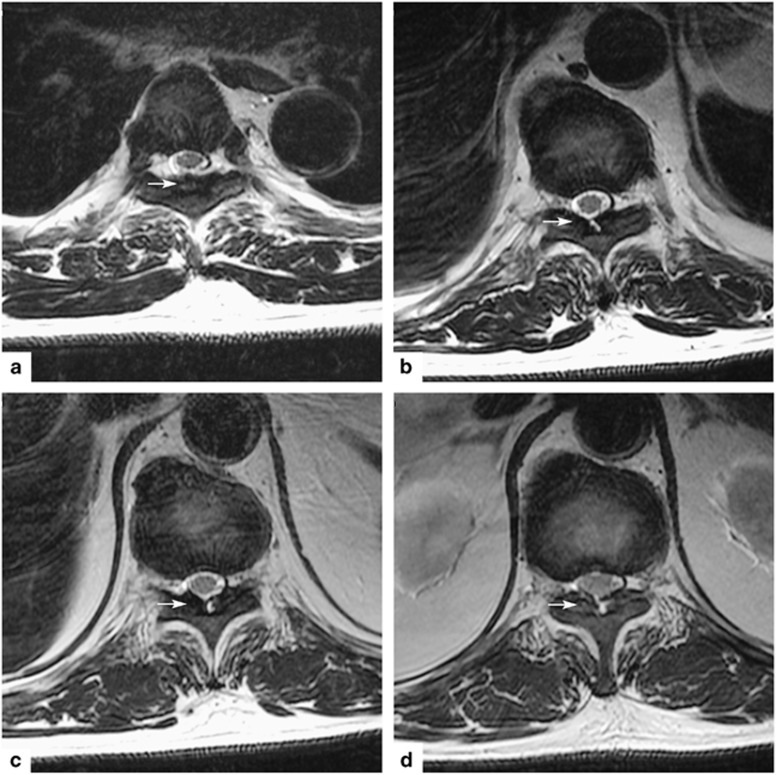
Axial images of hypointensity magnetic resonance imaging shows hypertrophy of the ligamentum flavum on T2-weighted image. (a) The lesion at the T3-T4 level. (b) The lesion at the T9-T10 level. (c) The lesion at the T10-T11 level. (d) The lesion at the T11-T12 level.
